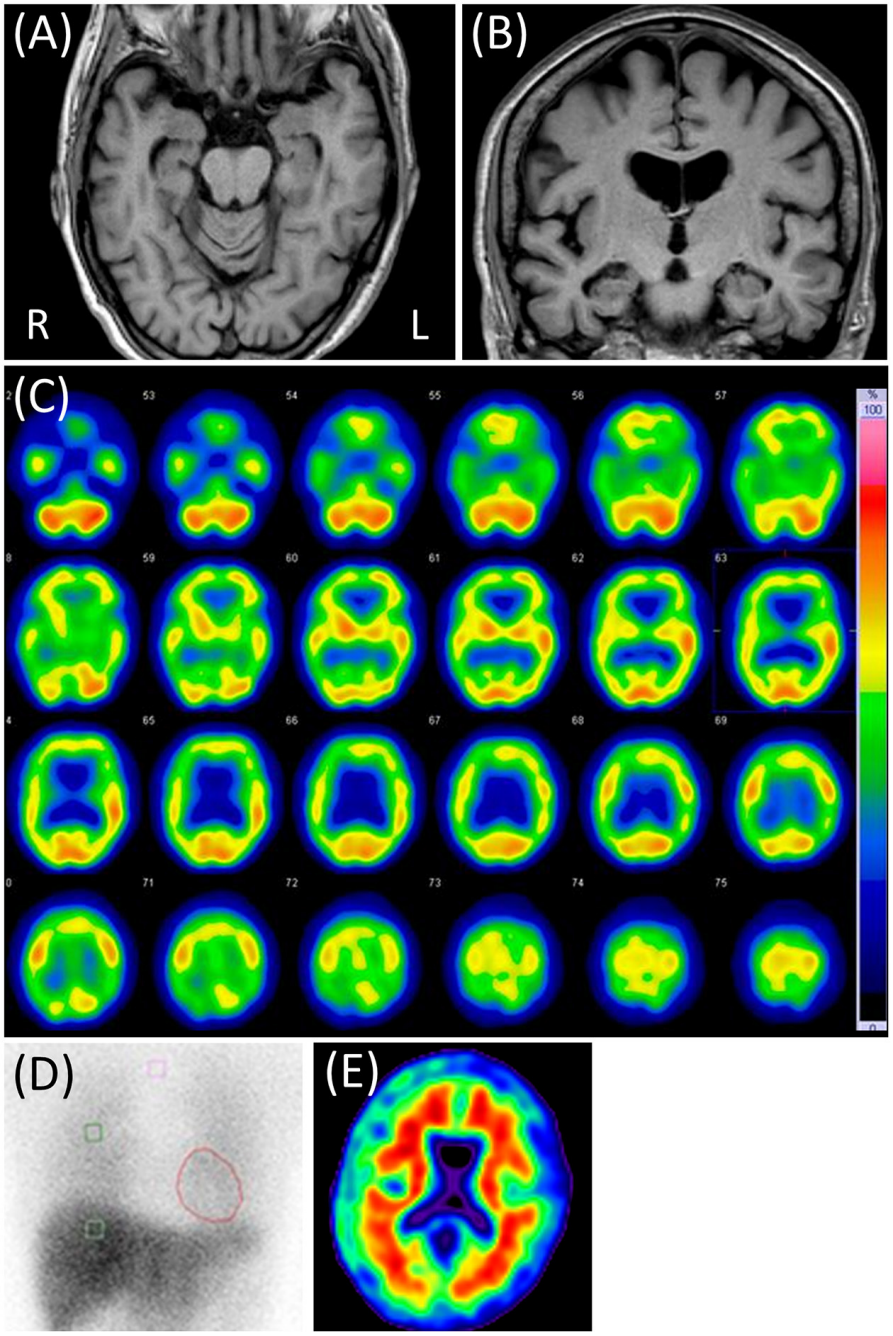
Case 2 images. (A,B) Brain magnetic resonance T1-weighted images showing slight diffuse brain atrophy. (C) N-isopropyl-p-[123I] iodoamphetamine single-photon emission computed tomography showing right-dominant hypoperfusion in the bilateral frontal, parietal, and temporal lobes. (D) Cardiac [123I]-metaiodobenzylguanidine scintigraphy. Heart-to-mediastinum ratios were 3.90 and 4.28 in early and delayed phases, respectively. The washout ratio was 26.0%. (E) Negative Florbetapir 18 positron emission tomography.
